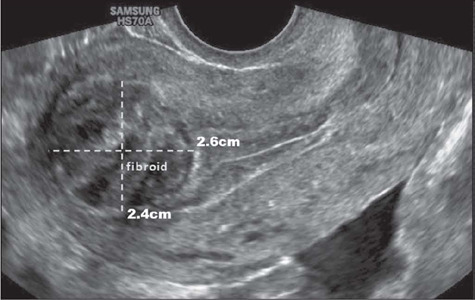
Transvaginal ultrasound image, in a longitudinal view, showing the dimensions of a
